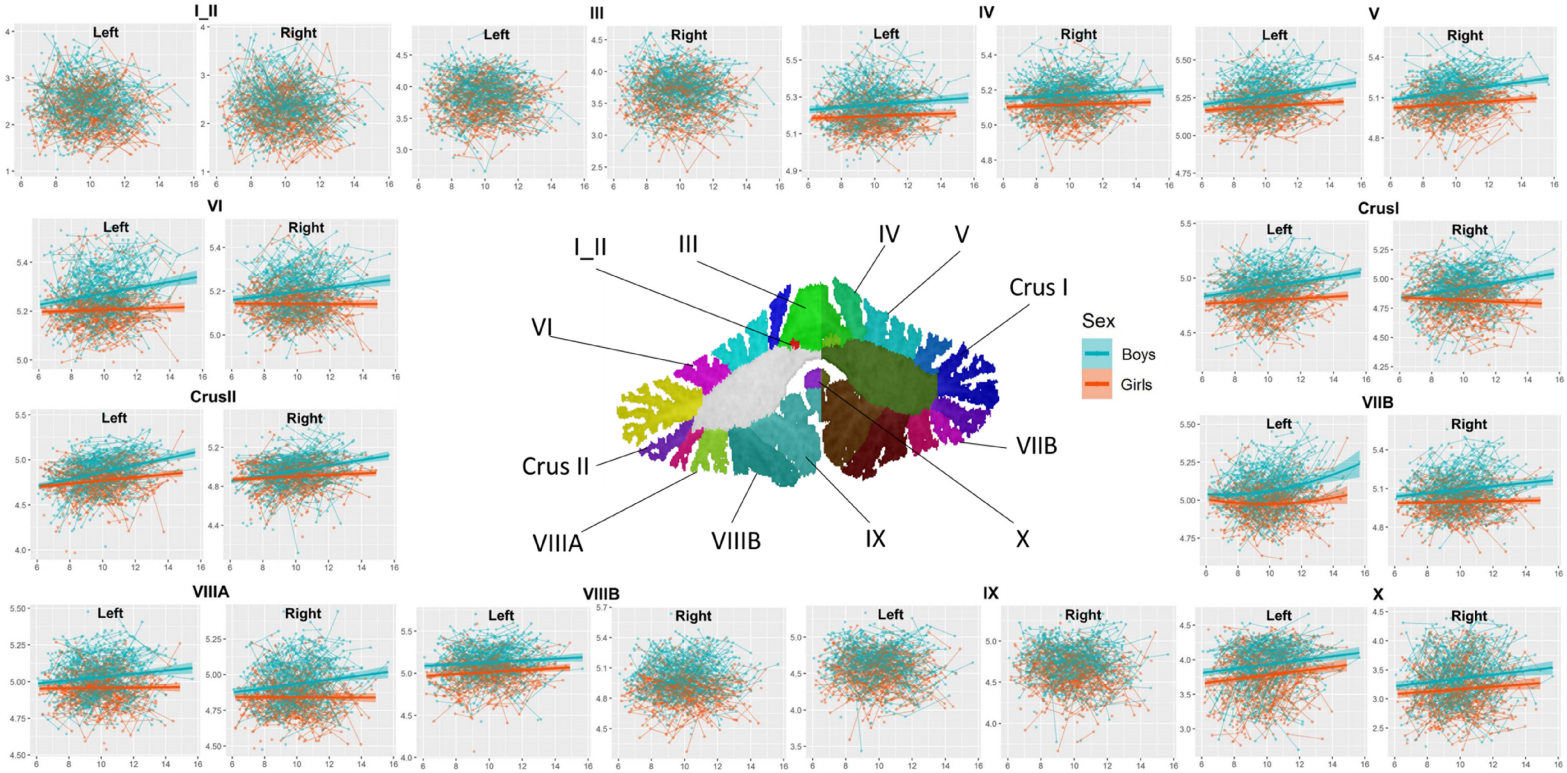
Development of CT in cerebellar subregions. CT (*y*‐axis) by age (*x*‐axis) and the optimal fitting models are shown. The shaded areas represent the 95% confidence intervals. Individual boys (blue) and girls (orange) are represented by individual lines, and participants measured once are represented by dots.
